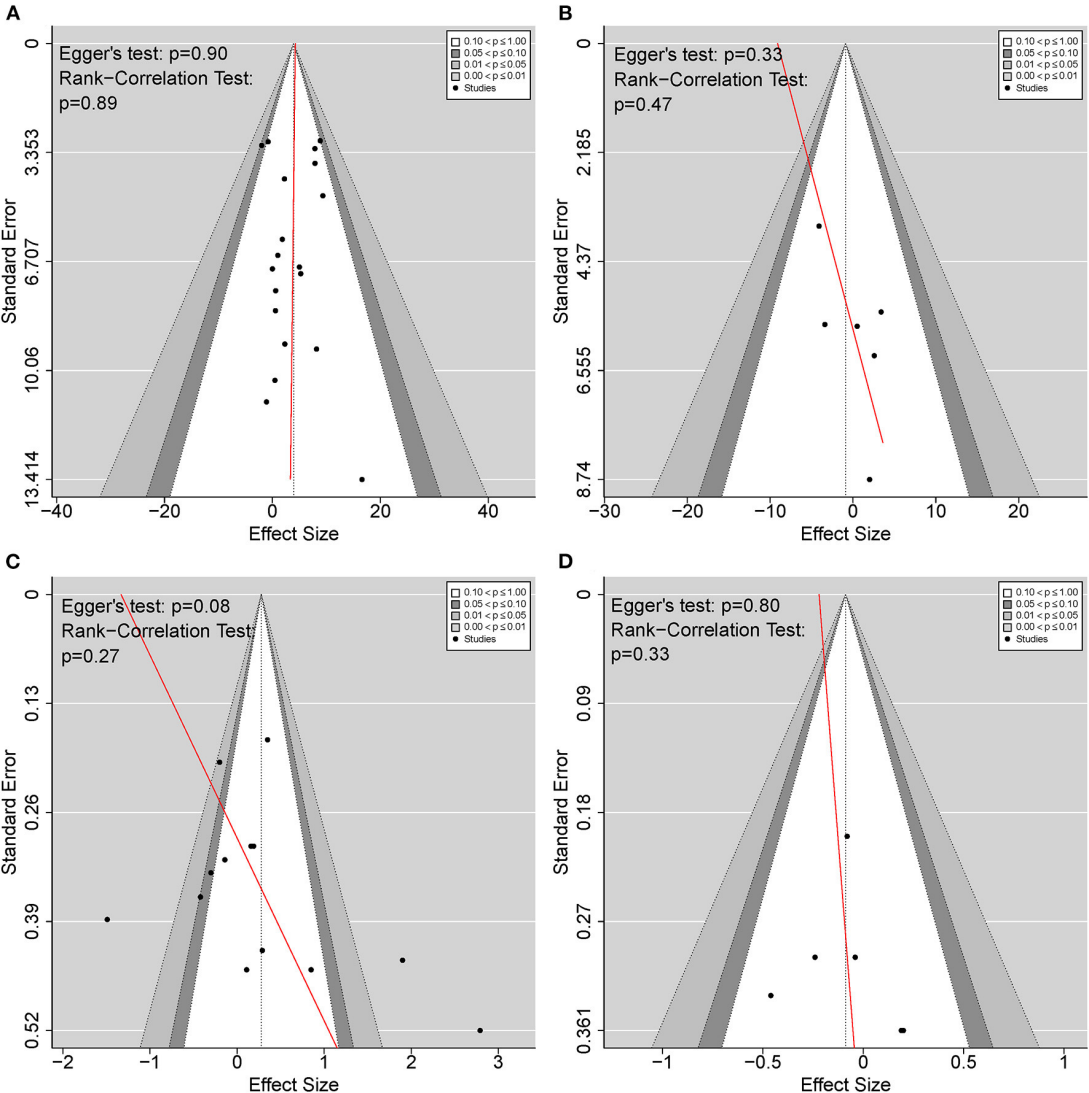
Funnel plots detecting publication bias. (A) BAT vs. CT on the upper extremity motor impairment; (B) BAT vs. UAT on the upper extremity motor impairment; (C) BAT vs. CT on the upper extremity functional performance; (D) BAT vs. UAT on the upper extremity functional performance. BAT, bilateral arm training; CT, conventional therapy; UAT, unilateral arm training.
